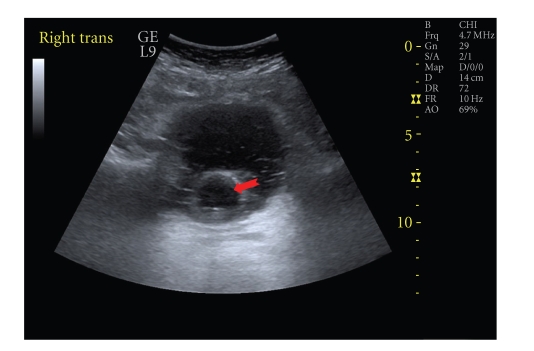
Follow-up ultrasound of urinary bladder after inserting a 16 French Foley catheter with 20 mL balloon. The balloon of Foley catheter was located within urinary bladder (arrow), thus confirming correct placement of Foley catheter.
